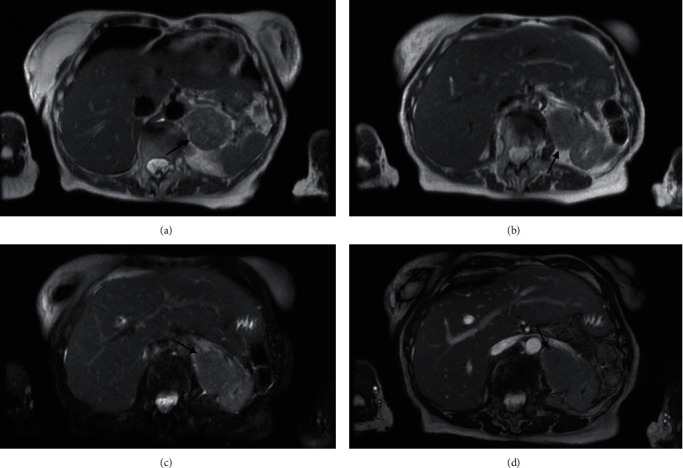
Follow-up MRI, ten months later, showed significant enlargement of the left adrenal mass, measuring 6.5 × 5.1 × 5.0 cm.
